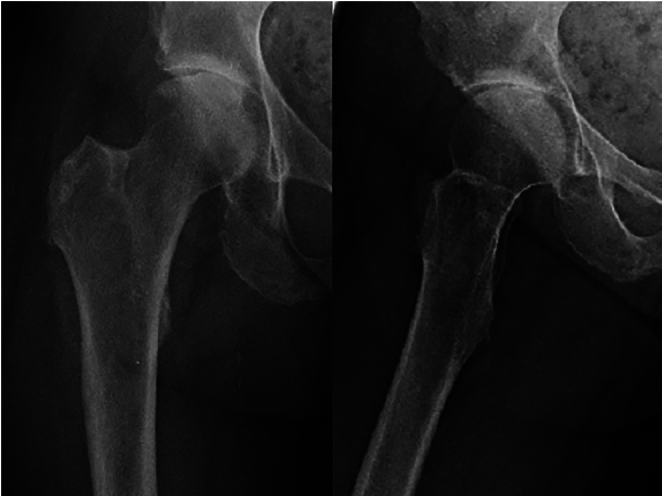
Preoperative X-rays showing arthritis of the right hip.
